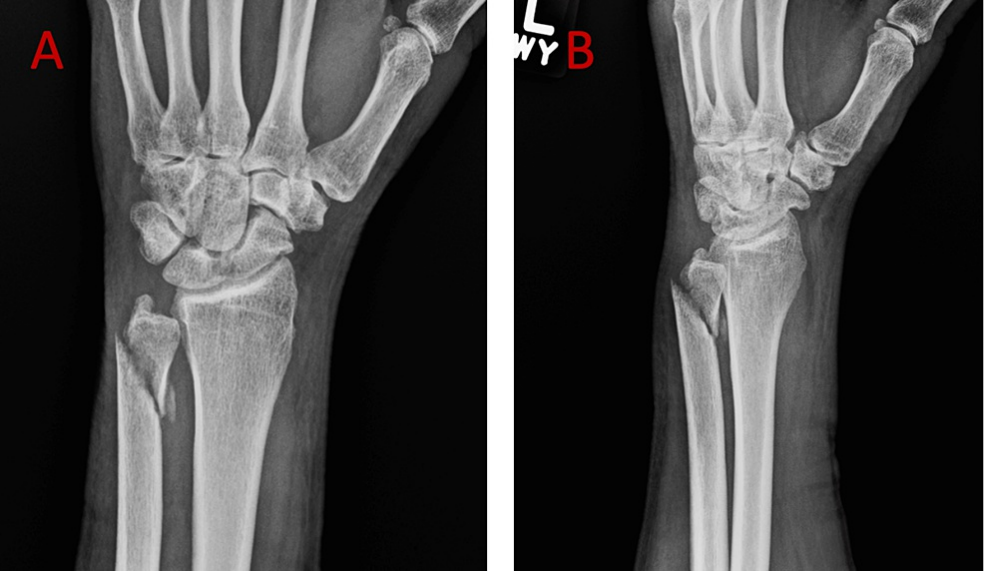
Anterior-posterior (A) and lateral (B) X-ray views of the left wrist 2-3 weeks post-injury showing interim displacement of the distal ulna fracture with a dorsal proximal bony spike.
